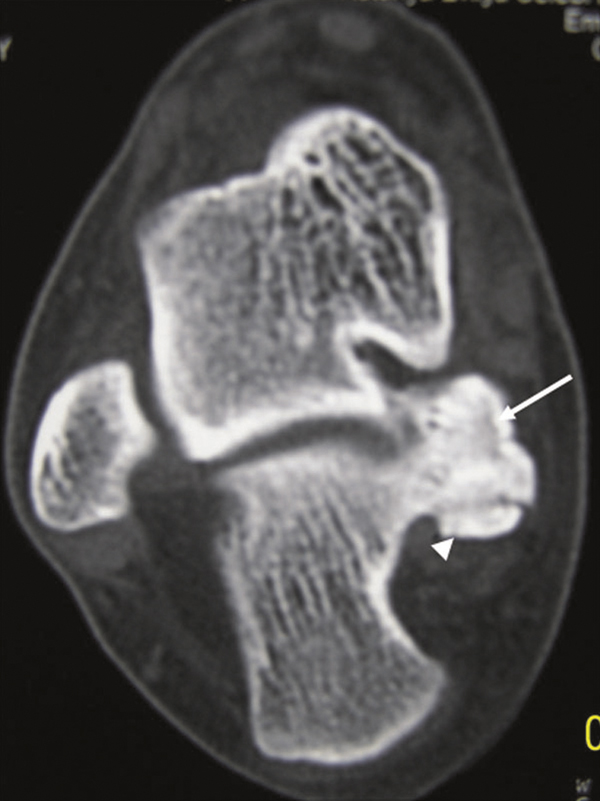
A preoperative axial CT scan of the foot shows the bone density lesion (arrow) raising from posteromedial of the calcaneus (27 × 23 mm in size, cartilage cap (arrowhead) thickness: 7 mm).
